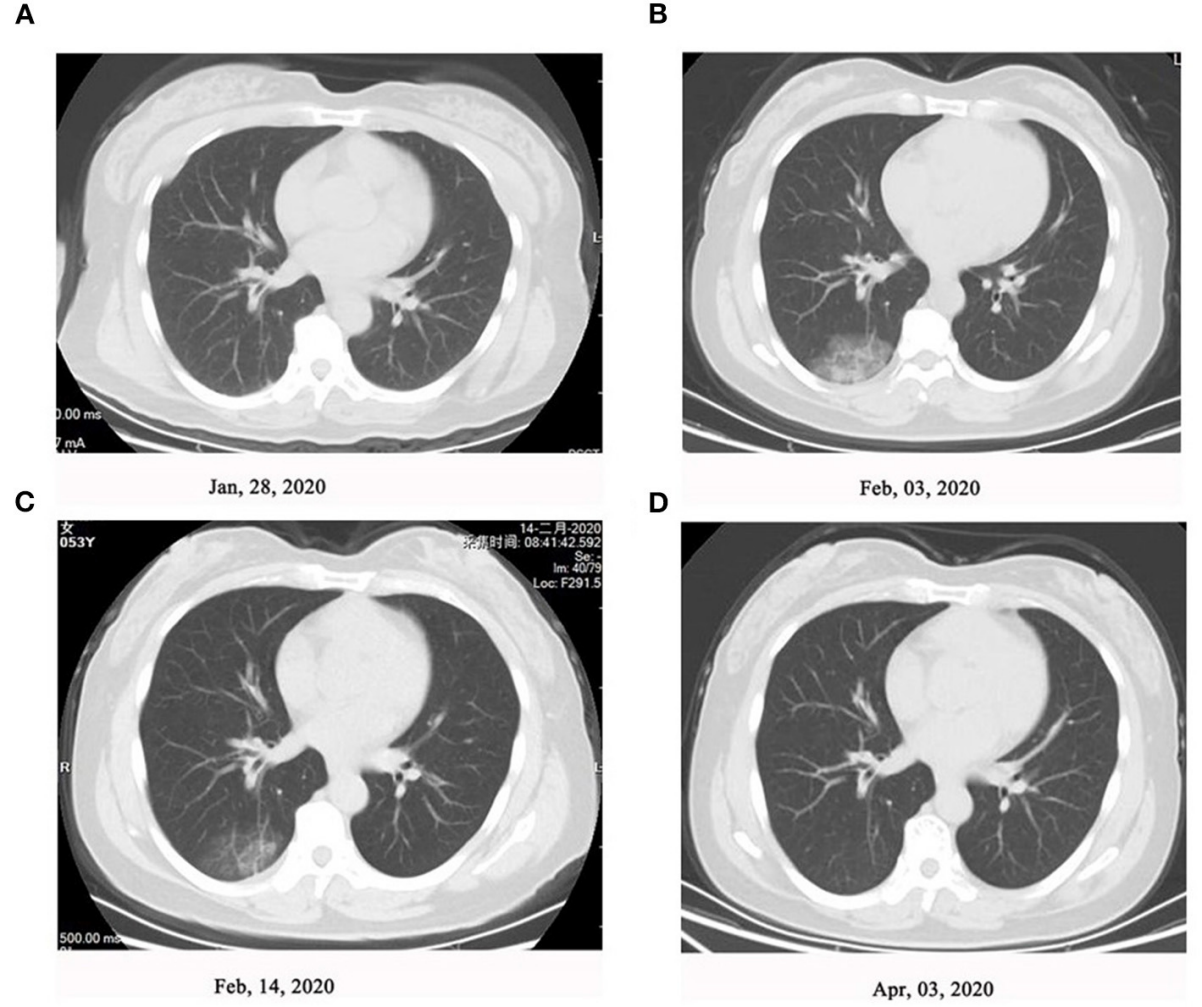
Representative changes of chest computed tomography (CT) images in Patient 3. High-resolution chest CT scan showed (A) normal chest at level of left atrium (January 28, 2020), (B) ground-glass attenuation close to the pleura in the right lower lung (February 03, 2020), (C) fade of ground-glass attenuation after treatment (February 14, 2020), and (D) resolution of lung inflammation after treatment (April 03, 2020).
